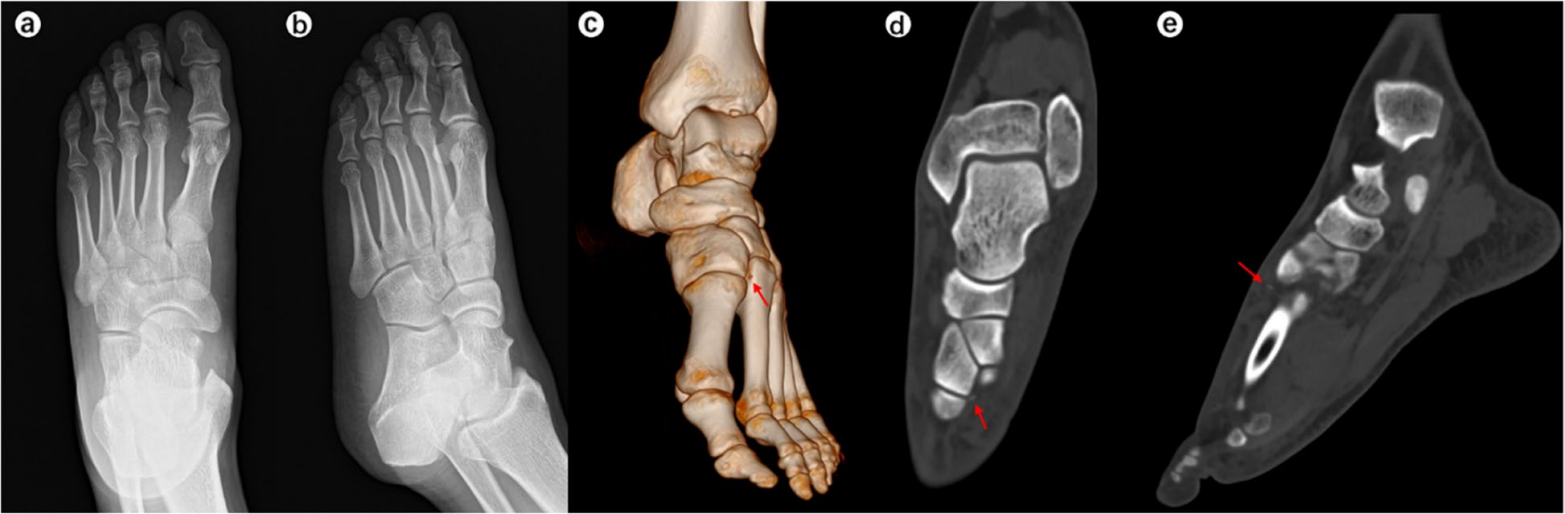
A 46-year-old man slipped and damaged his left foot. Chiodo-Myerson’s classification: medial-column injury; Displacement classification: non-displaced injury. Both two observers made the wrong diagnosis for two times, but didn’t change the initial treatment option (conservative treatment) after evaluating CT image. a-b The Lisfranc injury was missed on plain X-ray. c-e Computed tomography showed a dorsal avulsion fracture of the medial cuneiform bone. The red arrows indicate the very small fracture fragments
